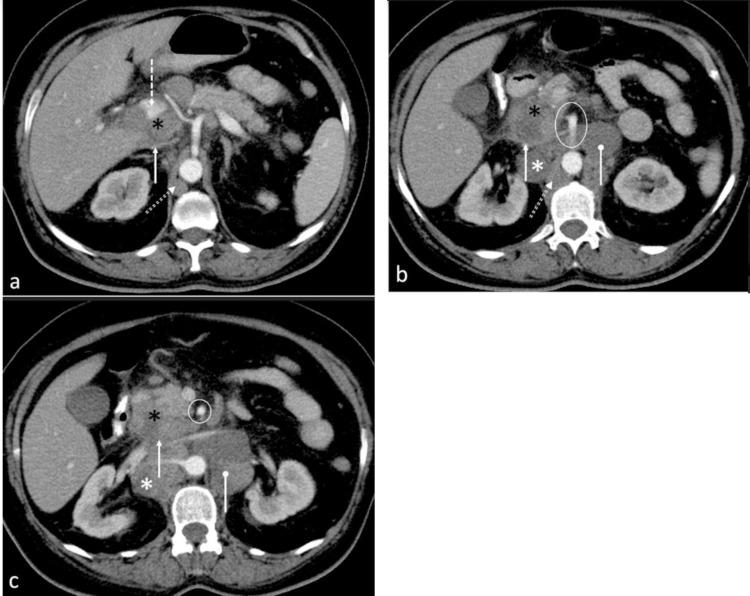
Contrast enhanced axial CT abdomen of a 43-year-old male at the level of the celiac artery origin (a) demonstrates an enlarged portacaval lymph node (black asterisk) which appears as a confluent soft tissue density mass between the portal vein (dotted white arrow) and IVC (solid white arrow). Images (b and c) acquired more caudally at and below the level of origin of the superior mesentery artery (circled) respectively, confirm compression of the IVC by lymph nodes. Note the enlarged retrocaval (white asterisk), para-aortic (oval arrow) and retrocrural (double dashed arrow) lymph nodes
